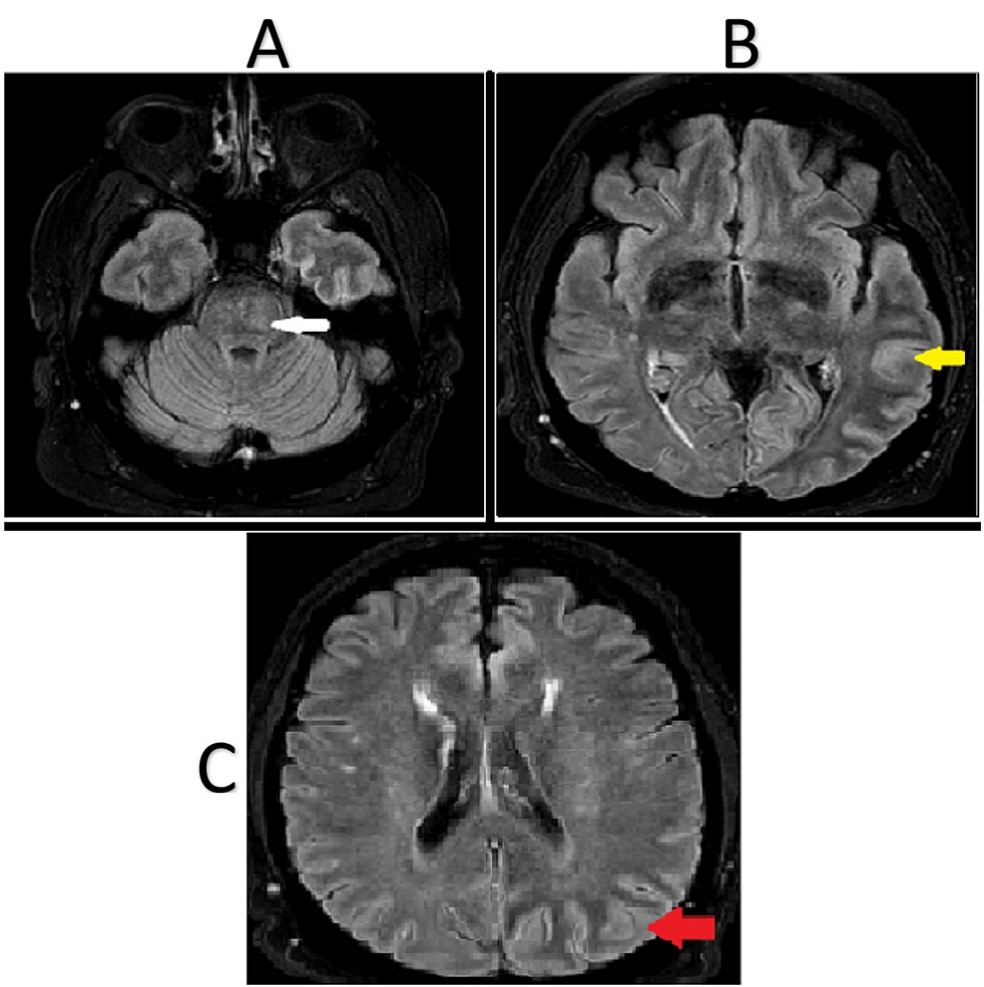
MRI brain showing increased FLAIR signal in the central pons, occipital, and temporal lobes. In image A, the white arrow highlights the increased FLAIR signal in the central pons. In image B, the yellow arrow depicts gyriform swelling and increased FLAIR signal in the left temporal lobe. In image C, the red arrow highlights gyriform swelling and increased FLAIR signal in the occipital lobe. MRI, magnetic resonance imaging; FLAIR, fluid-attenuated inversion recovery.
